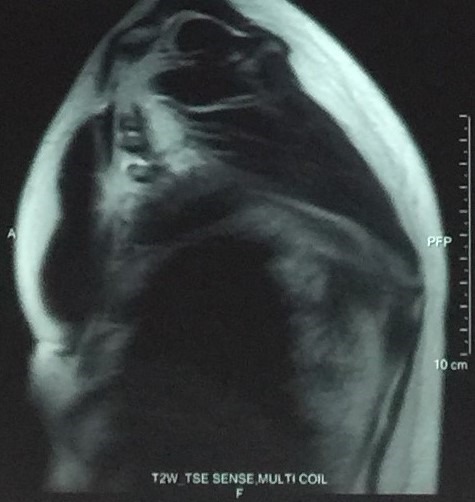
MRI image of elatofibroma dorsi (sagittal view)
